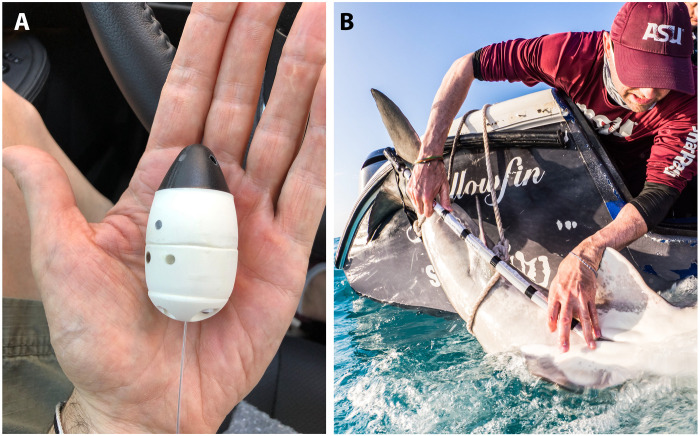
Image of BAT and applicator. (A) Representative image of the BAT. The egg-shaped device measures 6 cm in length by 2.5 cm in width and weighs 42 g in the air. (B) Insertion of the BAT via a specialized applicator into the cloacal opening of a gravid female tiger shark on 15 December 2019, also guided by simultaneous ultrasonography. Photo credit: T. Houppermans.
